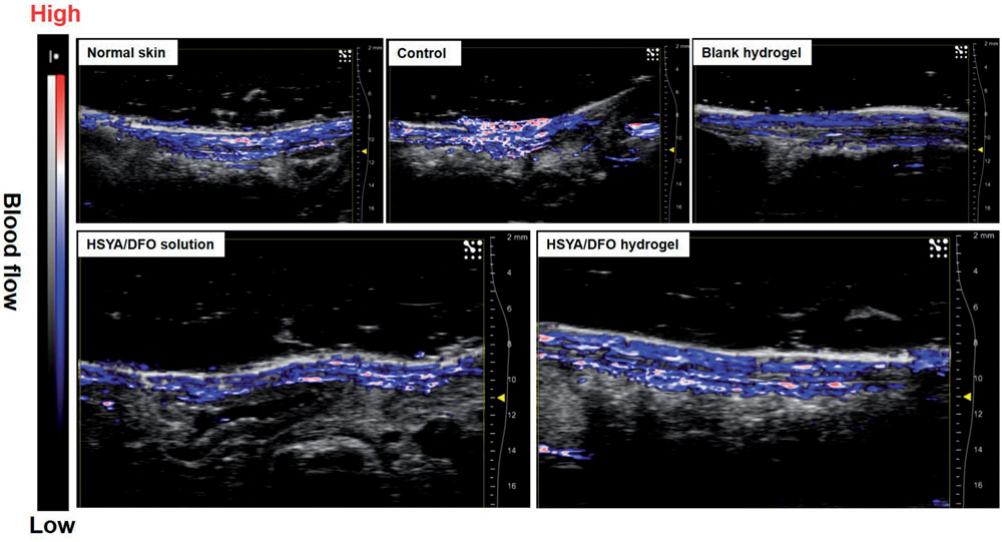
Cross-sectional combined ultrasound and photoacoustic images of diabetic wound for blood flow detection at day 28.
